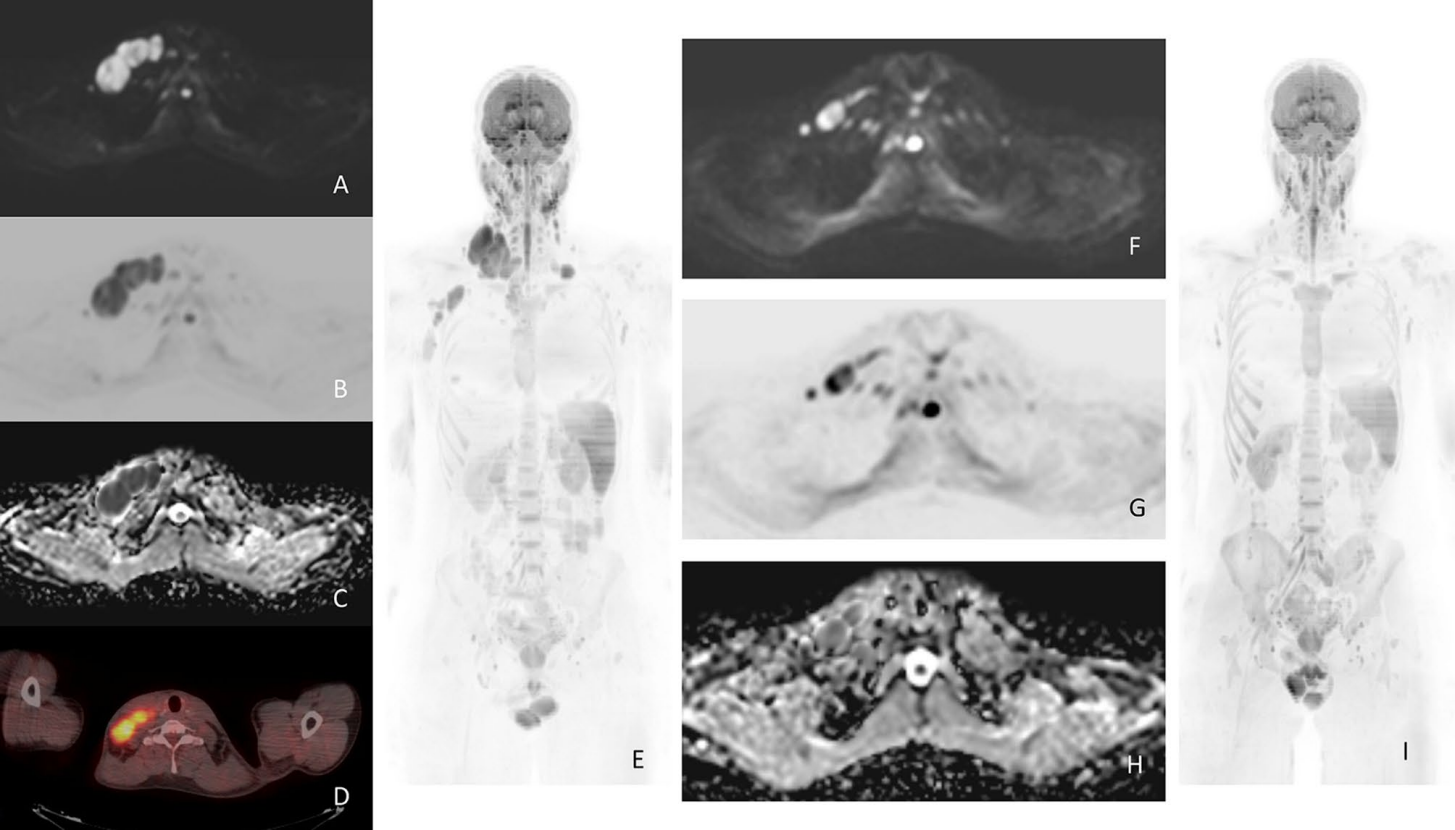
Baseline (A-E) and interim (F-I) Whole Body-MRI (WB-MRI) in a 31-year-old man with a mixed cellularity lymphoma and multiple confluent right supraclavicular nodal lesions at baseline that reduced in size at interim. At baseline, the lesions appear as hyperintense in the axial Diffusion Weighted image (b = 800 s/mm^2^) (A) and hypointense in the axial grayscale inverted Diffusion Weighted image (b = 800 s/mm^2^) (B). C On the baseline WB-MRI Apparent Diffusion Coefficient (ADC) map, the lesions appear as hypointense. D Baseline PET/CT shows large confluent nodal lesions in the same region with a high SUV value. E Coronal grayscale inverted MIP DW-MRI shows the same lesions that appear hypointense. At interim, the lesions appear significantly reduced in size and less hyperintense than baseline in the axial Diffusion Weighted image (b = 800 s/mm^2^) and (F) less hypointense than baseline in the axial grayscale inverted Diffusion Weighted image (b = 800 s/mm^2^) (G). H On the interim WB-MRI Apparent Diffusion Coefficient (ADC) map, the same lesions appear more hyperintense than baseline. I Coronal grayscale inverted MIP DW-MRI shows minimal residual disease
